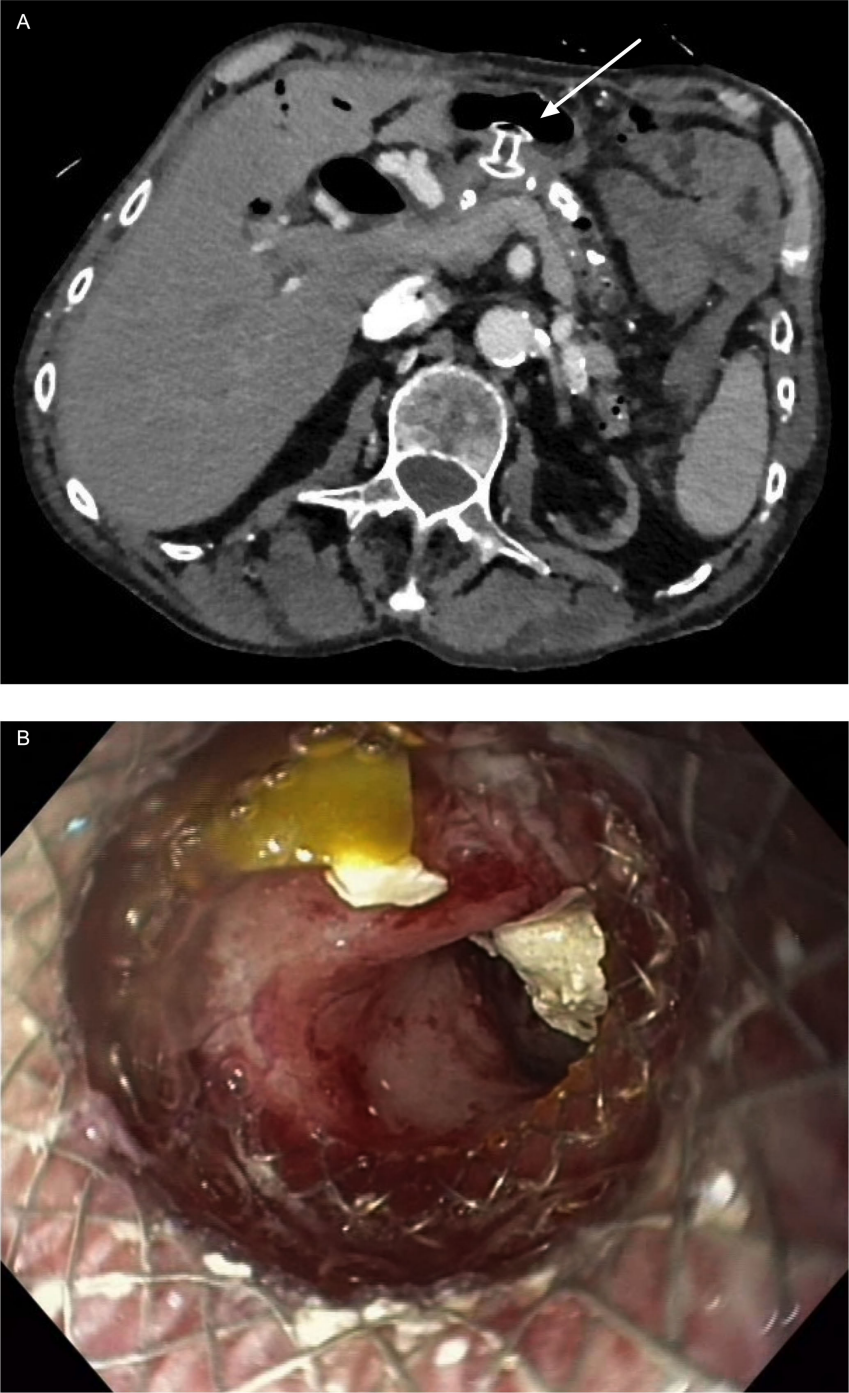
Case presentation of LAMS as gastro-pancreatostomy (HotAxios 8x8 mm, Boston Scientific, Marlborough, MA, USA): (A) CT scan showing LAMS in place in position of gastro-pancreatostomy; (B) transgastric view through LAMS into the pancreatic duct with residual stones fragments in the duct. (Pictures: Ellrichmann, Conrad).
